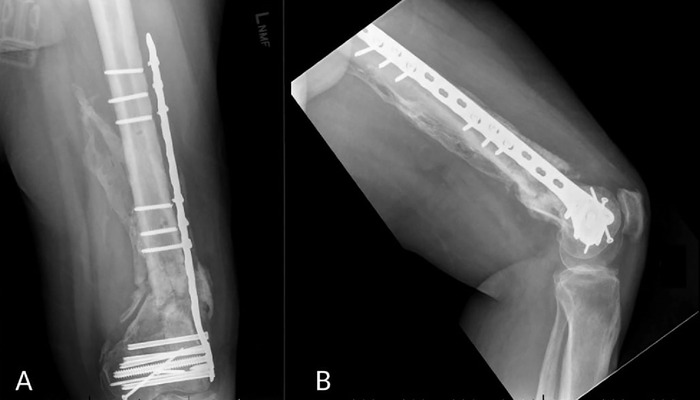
(A) Anteroposterior and (B) lateral radiographic films show hardware failure of the proximal plate with supracondylar nonunion occurring 16 months after the first open reduction and internal fixation procedure. This hardware failure was the first of five.
